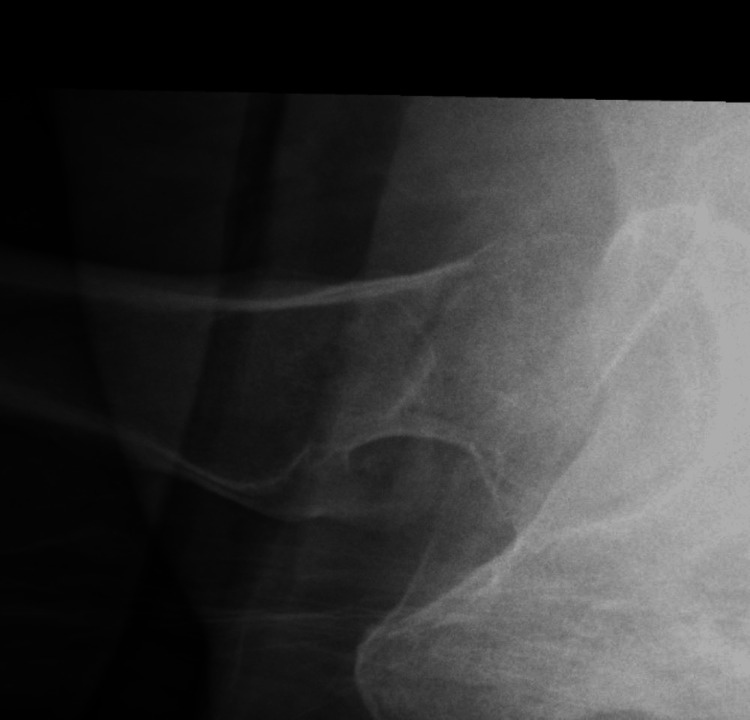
Lateral-view X-ray of the hip with NOF fracture NOF: neck of femur
